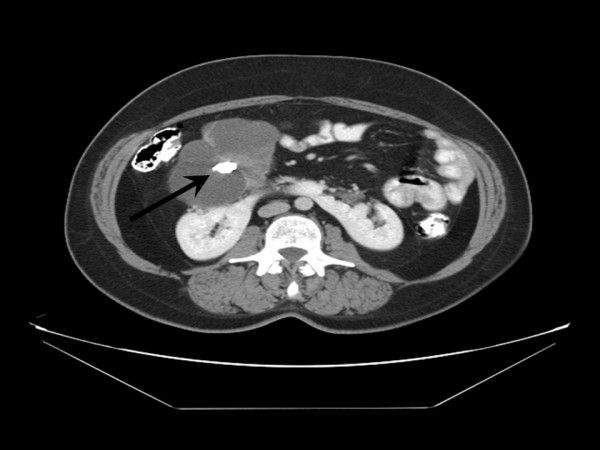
Computed tomography scan of abdomen and pelvis demonstrating horseshoe shaped kidney and a large multiseptated cystic lesion immediately anterior to the right renal pelvis with central calcification (arrow).
